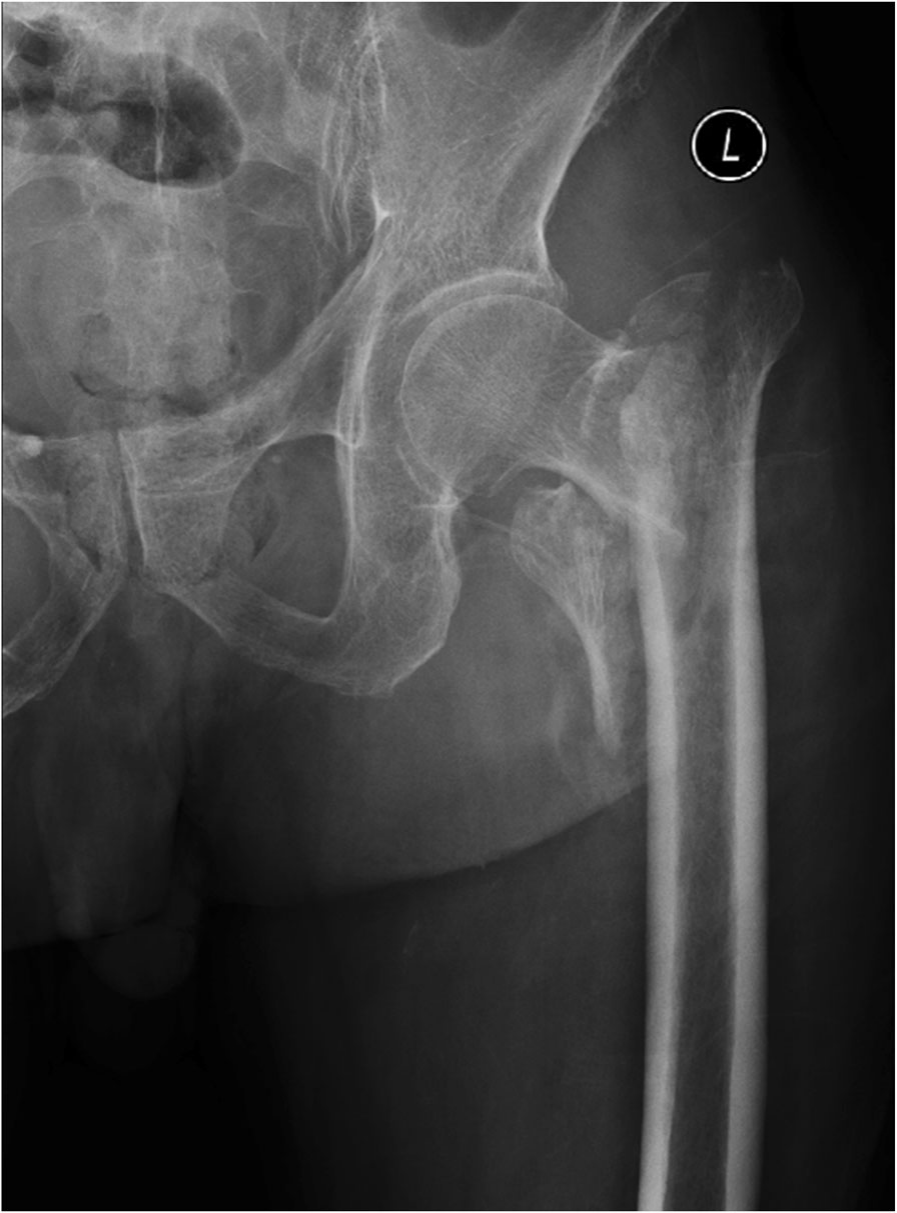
X-ray indicated left intertrochanteric fracture
